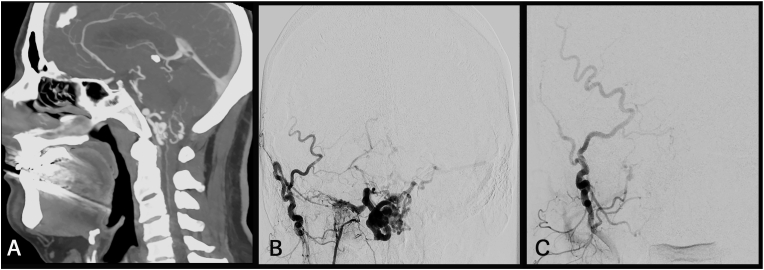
(A) Preoperative CTA displaying a foramen magnum fistula with enlarged veins within the posterior fossa. (B) Preoperative angiogram demonstrating the AV-fistula with feeders from the external carotid artery (ECA) in anterior posterior (A.P.) projection. (C) Postoperative digital subtraction angiography (DSA) depicting complete occlusion of the fistula.
